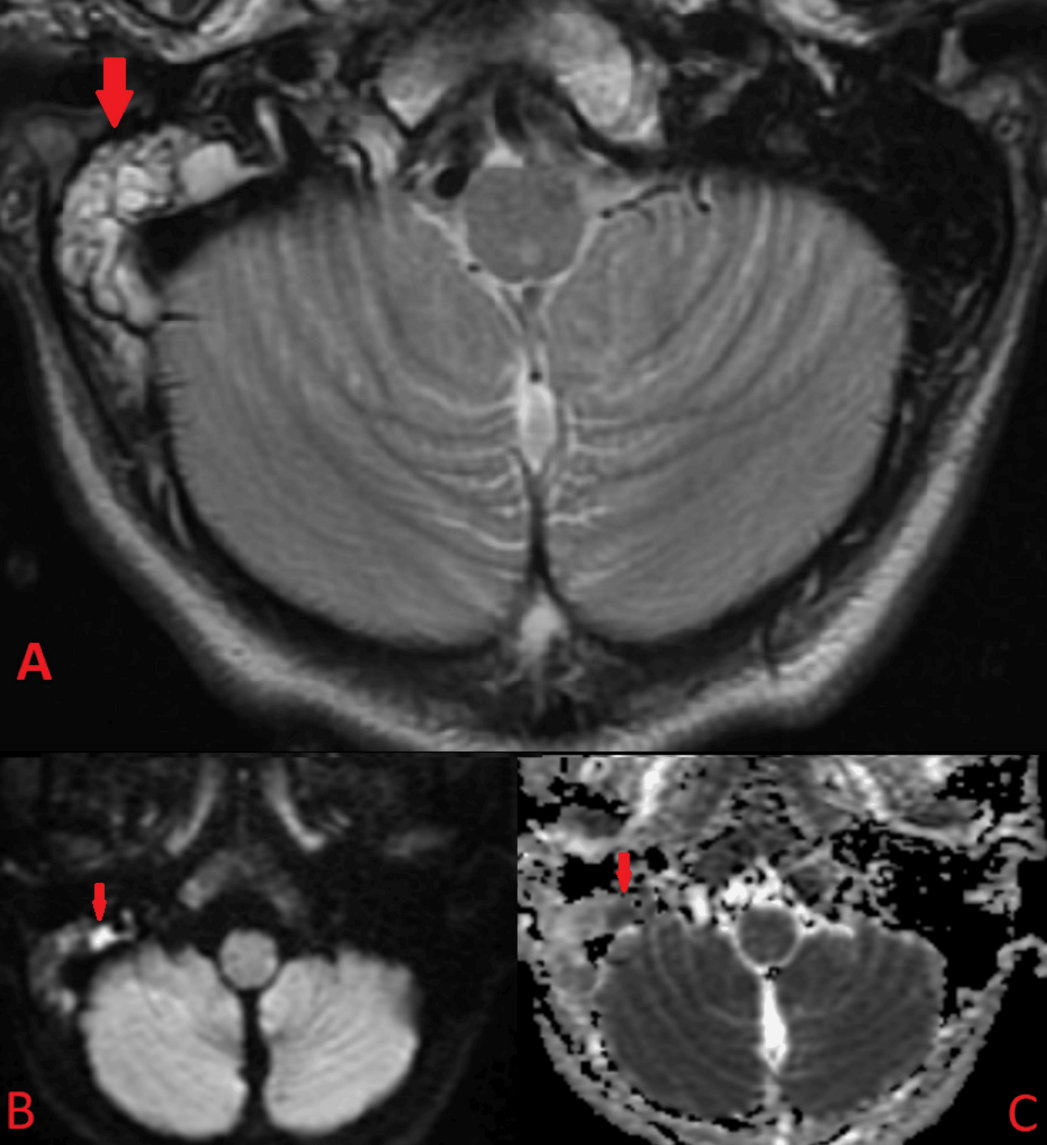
MRI brain axial T2 fast spin echo (A), axial DWI (B), and axial ADC (C) at the level of the mastoid air cells and posterior fossa. There is an increased T2 signal within the right mastoid air cells (red arrows) consistent with complete opacification. On corresponding DWI and ADC, there is diffusion restriction likely secondary to infection/pus. This is highly suggestive of mastoiditis in the correct clinical setting.
